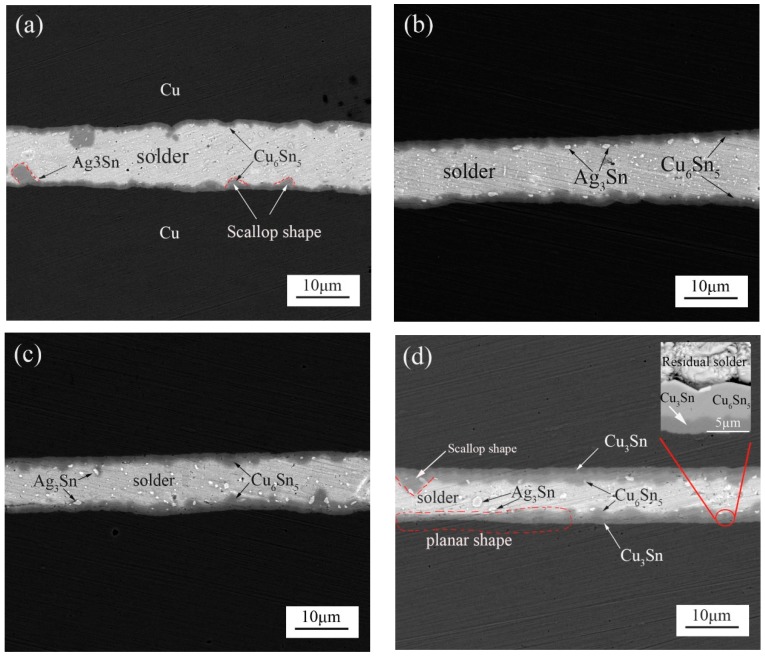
The morphologies of the cross-sectional Cu/SAC305/Cu joints formed at a 400-ms ultrasonic bonding time at different aging times of (a) 0 h; (b) 120 h; (c) 240 h and (d) 360 h. The inset in (d): the local enlargement image at the interfaces.
